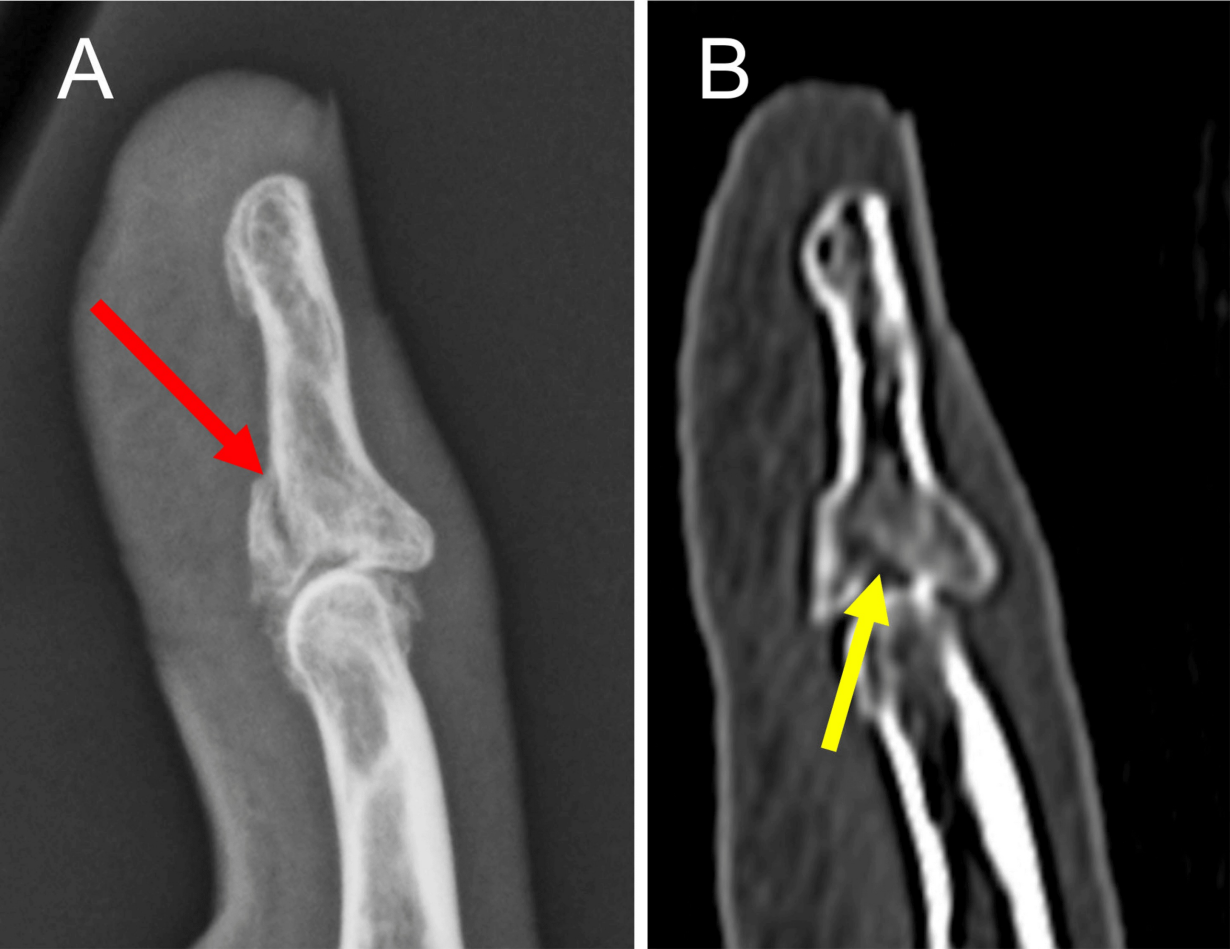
Preoperative radiograph and CT scan of Patient 1 The radiograph shows a split-depression type fracture (red arrow) with impaction of the radial articular surface in the lateral view of the right ring finger (A). The sagittal view shows an impaction of the volar fragment especially on the radial side (yellow arrow) with dorsal subluxation of the DIP joint (B). DIP: distal interphalangeal
